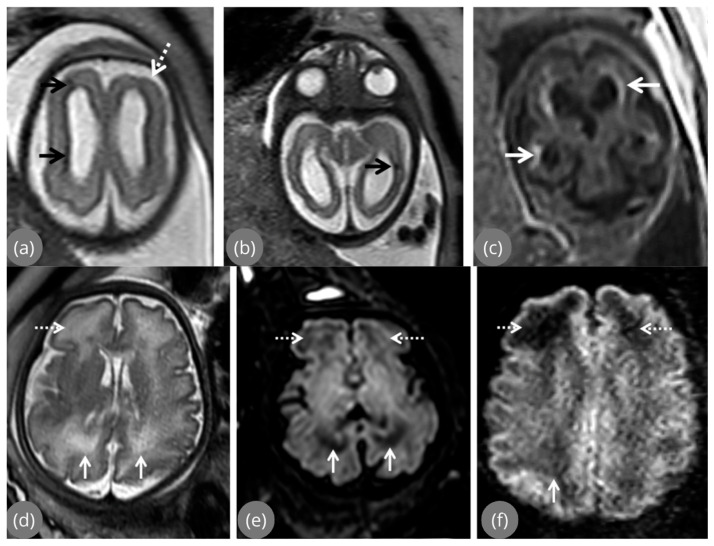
MRI features typical of CMV infection [13]. (a,b) focal signal anomaly—black arrows, (a) frontal polymicrogyria—white dashed arrow, (c) calcifications—white arrows, (d) white matter hyperintensities in the frontal—white dashed arrows and parieto-occipital region—white arrows, (e) T2w echo planar imaging-FLAIR hypointensity—white/dashed arrows, and (f) low SI on the zoom diffusion weighted image—white/dashed arrows.
